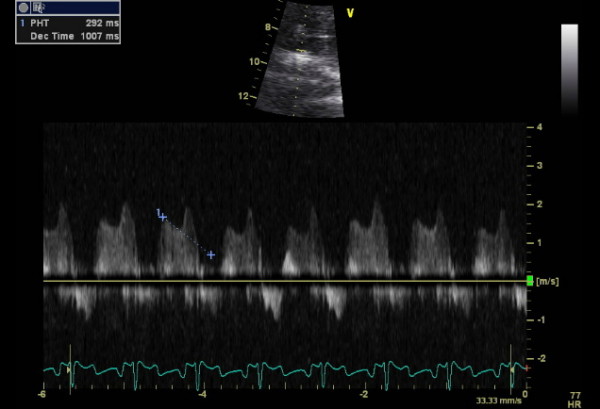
Doppler echocardiogram showing a prolonged pressure half time of 270 msec suggestive of severe prosthetic tricuspid obstruction.
